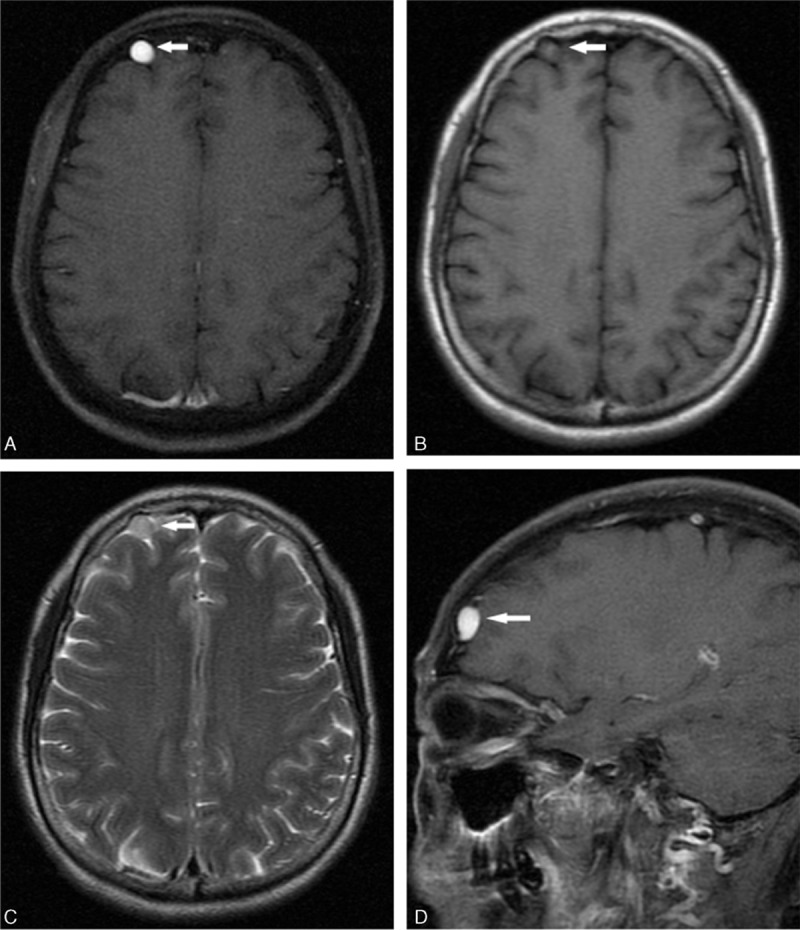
Preoperative magnetic resonance images postcontrast images revealed a rounded lesion on the axial T1-weighted image (arrow, A) and the spindle-shaped on sagittal image (arrow, D), with homogeneous-marked enhancement in the right frontal convexity. It is isointense with gray matter on the T1-wighted image (arrow, B) and had slightly hyperintensity on the T2-wighted image (arrow, C).
